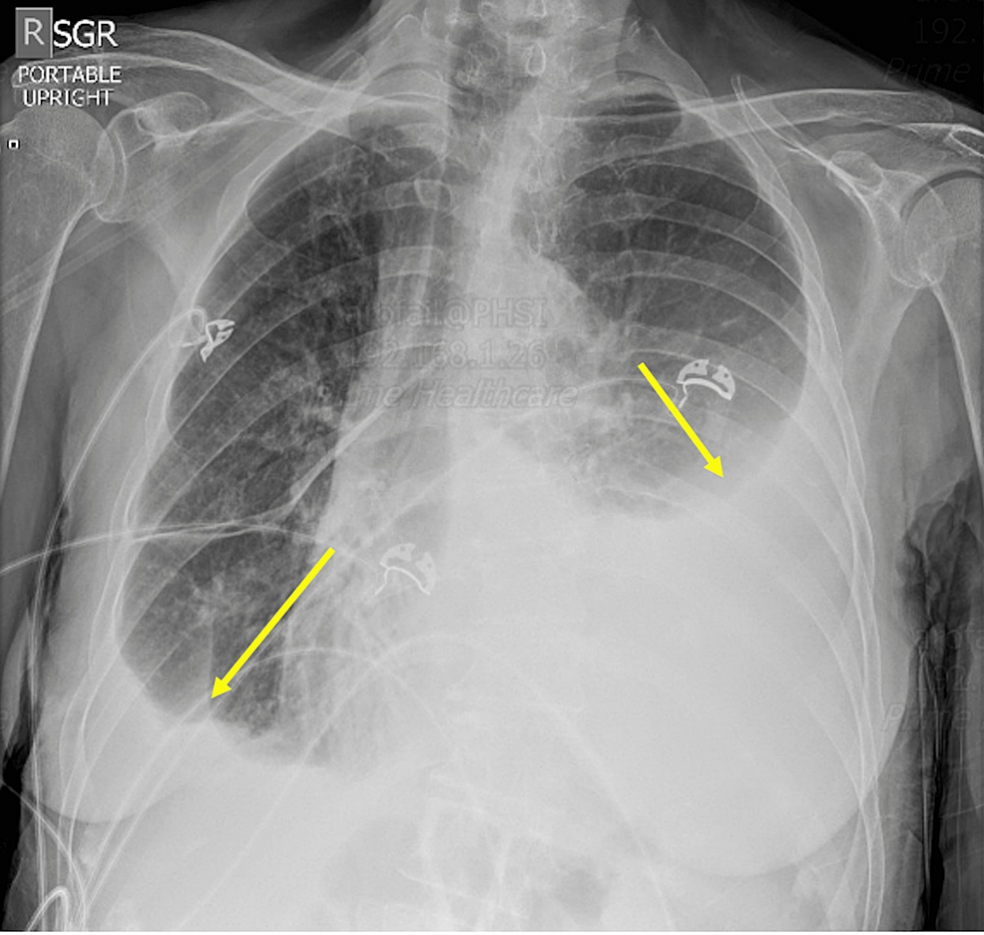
Chest X-ray showing bilateral pleural effusions.
